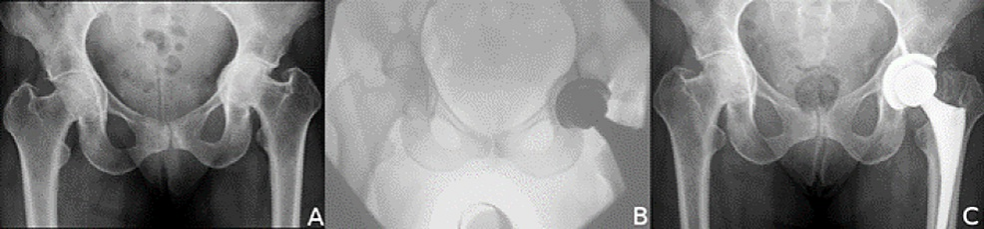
Protrusio acetabuli managed with the direct anterior approach and intraoperative fluoroscopy. (A) Preoperative anteroposterior (AP) pelvic radiograph with typical protrusio deformities. (B) AP intraoperative image to assess medial graft, cup orientation, and leg length. (C) Six-year follow-up AP pelvic radiograph with consolidation of bone graft, no radiolucent lines, restoration of offset, and correction of leg length discrepancy.
